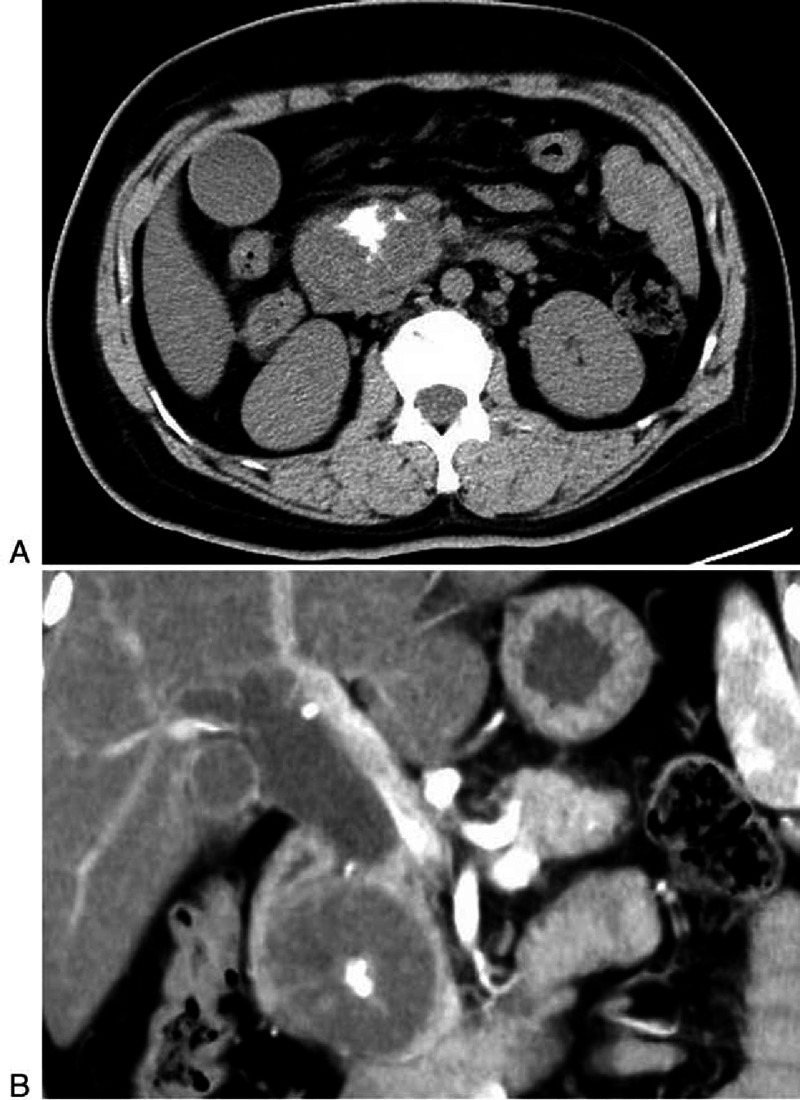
A 38-year-old woman with osteosarcoma (patient 16). A, Unenhanced CT demonstrate the lesion in the pancreatic head presents cystic lesion, with calcification and partition inside the tumor. B, Coronal multiplanar reconstruction from contrast enhanced CT show evident dilation of common bile duct as a result of being oppressed by the well-defined mass.
